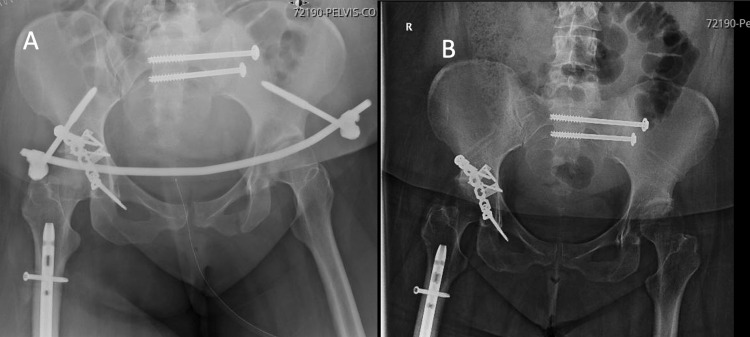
Stabilization of the combined pelvic ring and acetabulum fracture in Figure 4 began with posterior fixation first followed by flexible anterior fixation utilizing an INFIX and finalized with acetabular stabilization through a posterior Kocher-Langenbeck approach (A). Final x-rays after INFIX removal (B).
